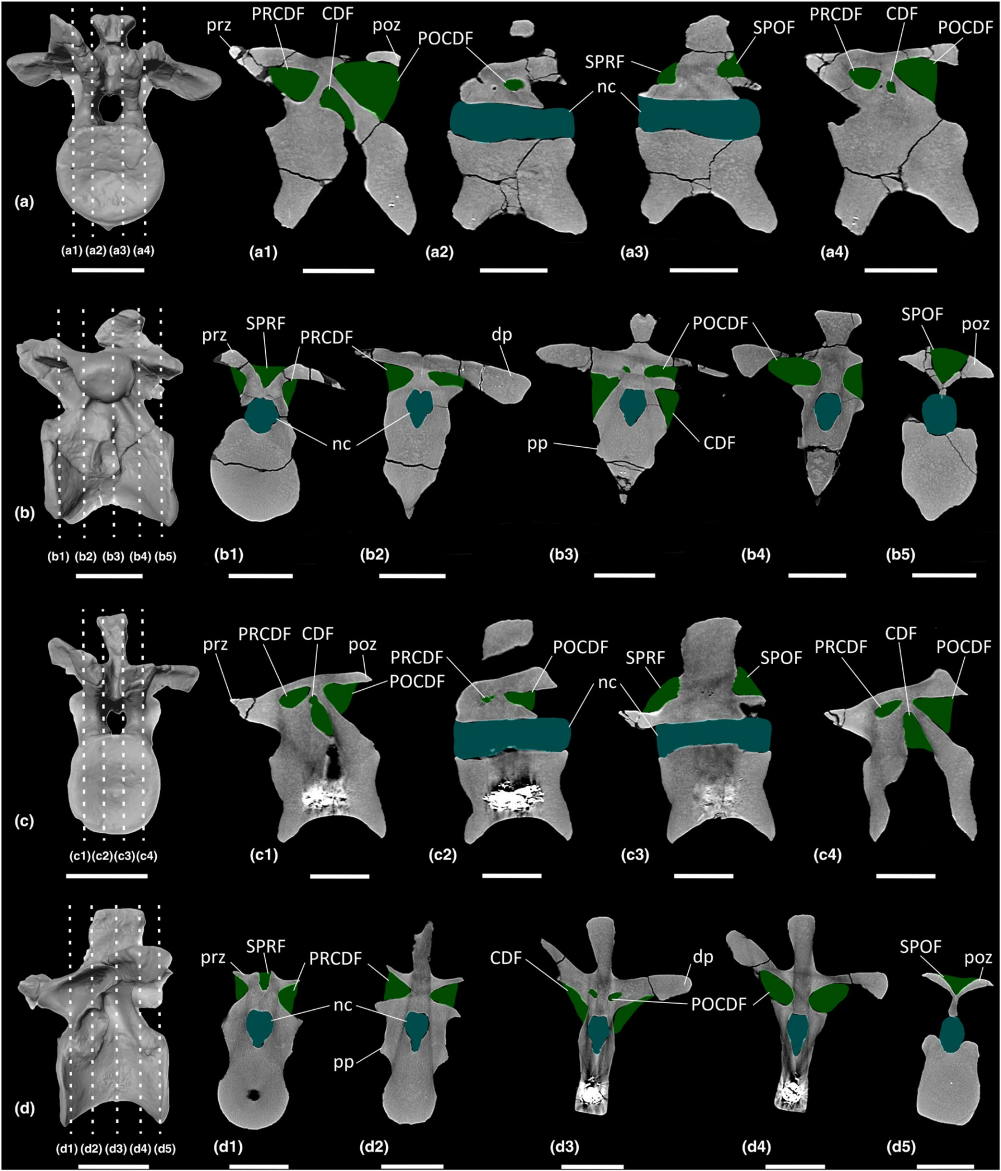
CT scan data of *Ruehleia bedheimensis* dorsal vertebrae. (a, b) dorsal vertebra 1 MB.R.4718.41, (c, d) dorsal vertebra 5 MB.R.4718.45; (a, c) surface scan anterior view, (b, d) surface scan lateral view; (a1–a4, c1–c4) CT scan slices in parasagittal cross‐section, (b1–b5, d1–d5) CT scan slices in transverse cross‐section. CDF, centrodiapophyseal fossa; dp, diapophysis; nc, neural canal; POCDF, postzygapophyseal centrodiapophyseal fossa; poz, postzygapophysis; pp, parapophysis; PRCDF, prezygapophyseal centrodiapophyseal fossa; prz, prezygapophysis; SPOF, spinopostzygapophyseal fossa; SPRF, spinoprezygapophyseal fossa. Key: Blue highlight, neural canal; green highlight, fossa. Scale bars = 5 cm.
